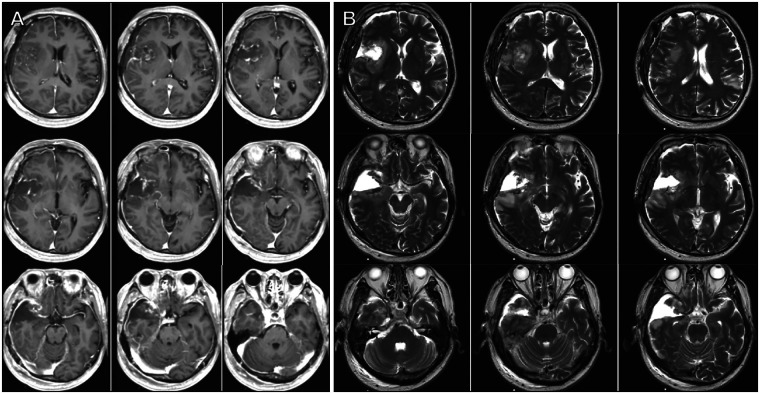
Postoperative image acquisition. Postoperative MRI at 48 h revealed complete resection of the enhanced lesion [(A), T1CE], with no evidence of complications such as significant hemorrhage or cerebral infarction in the surgical area [(B), T2WI].
